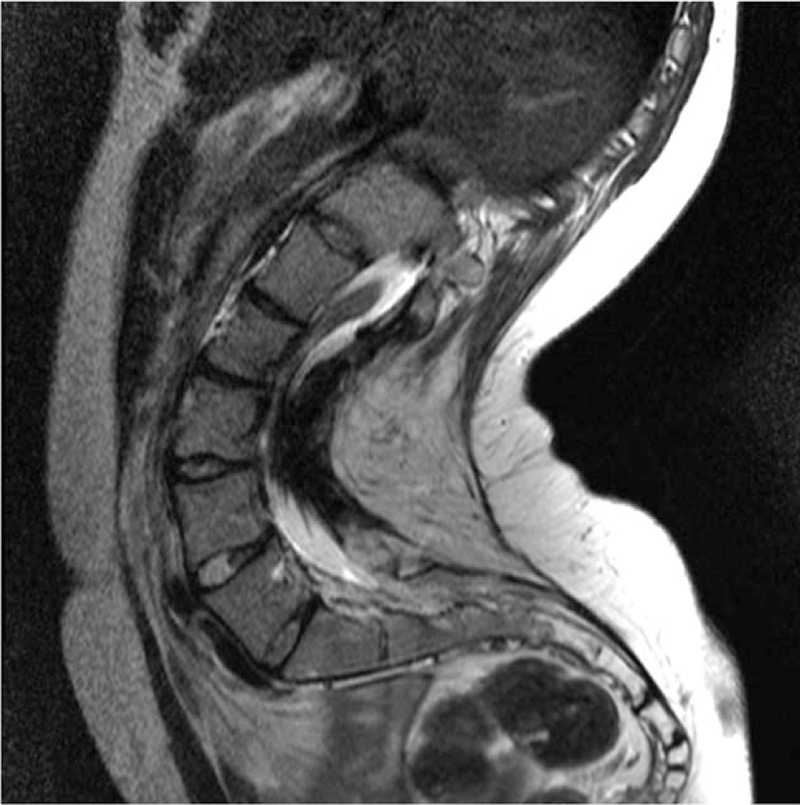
Preoperative magnetic resonance imaging showing no abnormality in the spinal canal.
